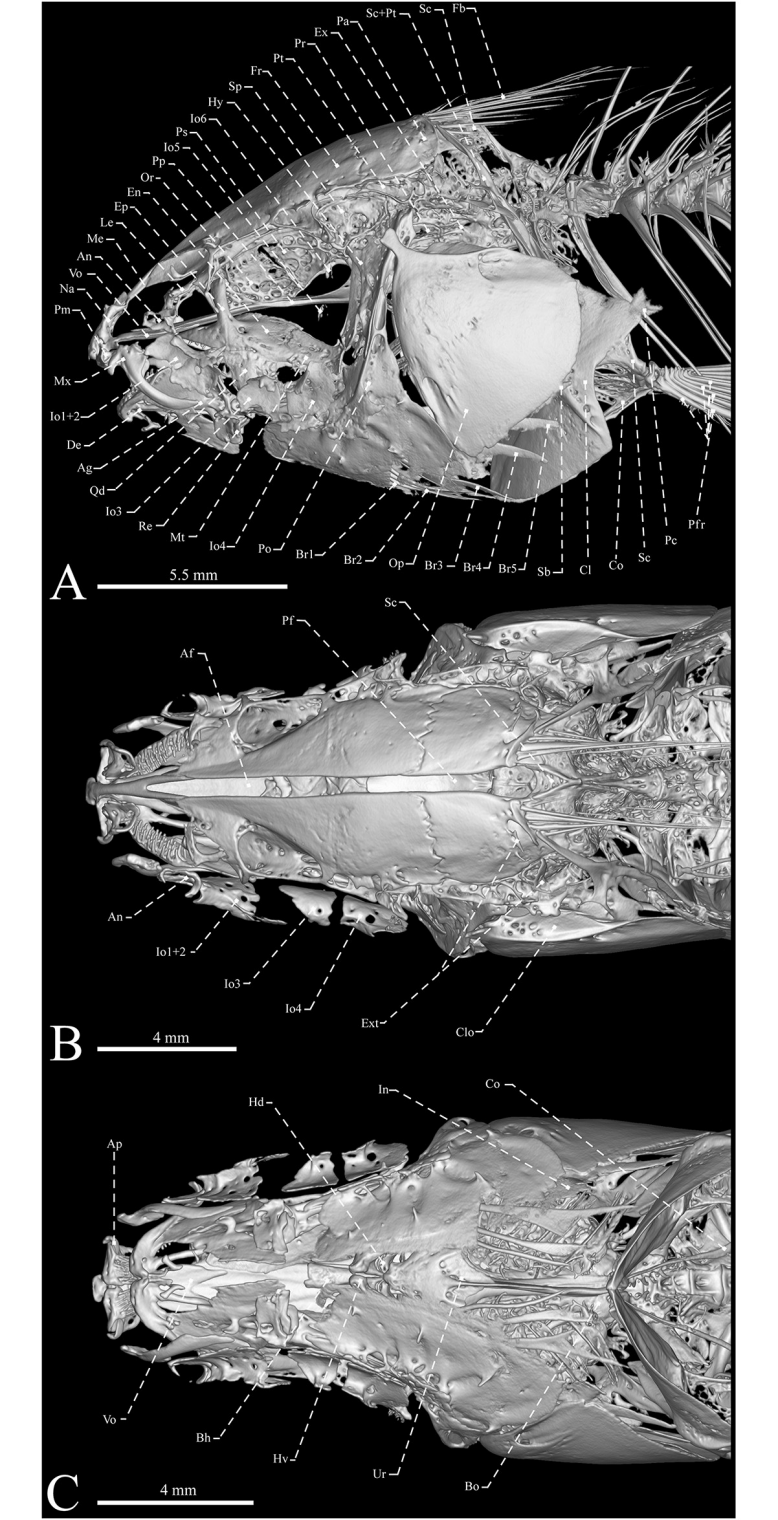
Computed tomography renderings of anterior portion in lateral (A), dorsal (B) and ventral views (C) of *Eigenmannia sirius*, MZSUP 121668, holotype, 127.5 mm *L*_EA_. Af = anterior fontanelle; Ag = anguloarticular; Ao = antorbital; Ap = anterolateral process of premaxilla; Bh = basihyal; Bo = basioccipital; Br = branchiostegal rays; Cl = Cleithrum; Clo = crest of the *levator arcus palatini*; Co = coracoid; De = dentary; En = endopterygoid; Ep = endopterygoid process; Ex = exoccipital; Ext = extrascapular; Fb = filamentous bone; Fr = frontal; Hd = hypohyal dorsal; Hv = hypohyahl ventral; Hy = hyomandibula; In = Interopercle; Io = infraorbitals; Le = lateral ethmoid; Me = mesethmoid; Mt = metapterygoid; Mx = maxillary bone; Na = nasal; Op = opercle; Or = orbtosphenoid; Pa = parietal; Pc = postcleithrum; Pfr = pectoral-fin rays; Pf = posterior fontanelle; Pm = premaxilla; Po = preopercle; Pp = parasphenoid; Pr = prootic; Ps = pterosphenoid; Pt = pterotic; Qd = quadrate; Re = retroarticular; So = subopercle; Sca = scapula; Sc+Pt = suptracleithrum+posttemporal; So = supraoccipital; Sp = sphenotic; Ur = urohyal; Vo = vomer.
